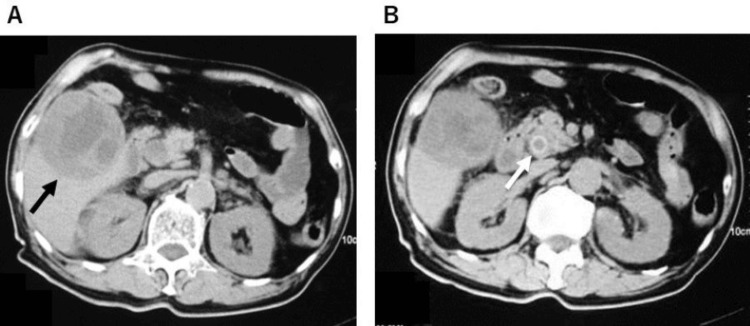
Pretreatment computed tomography images. (A) A significantly thickened gallbladder wall containing fluid collection (black arrow) and (B) incarceration of gallstone in the common bile duct (white arrow).
